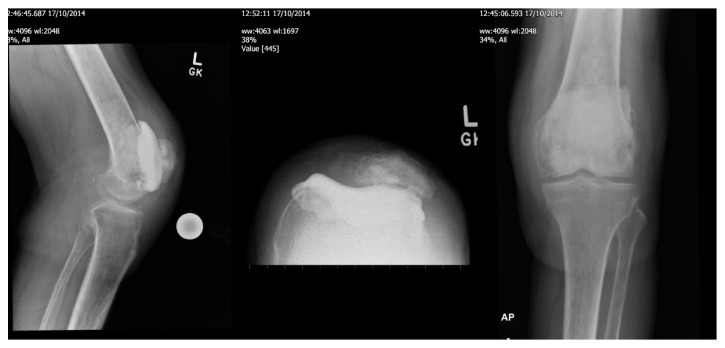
Postoperative radiograph of 1st stage revision with cement spacer, also seen is the cystic lesion on femoral side packed with bone graft.
